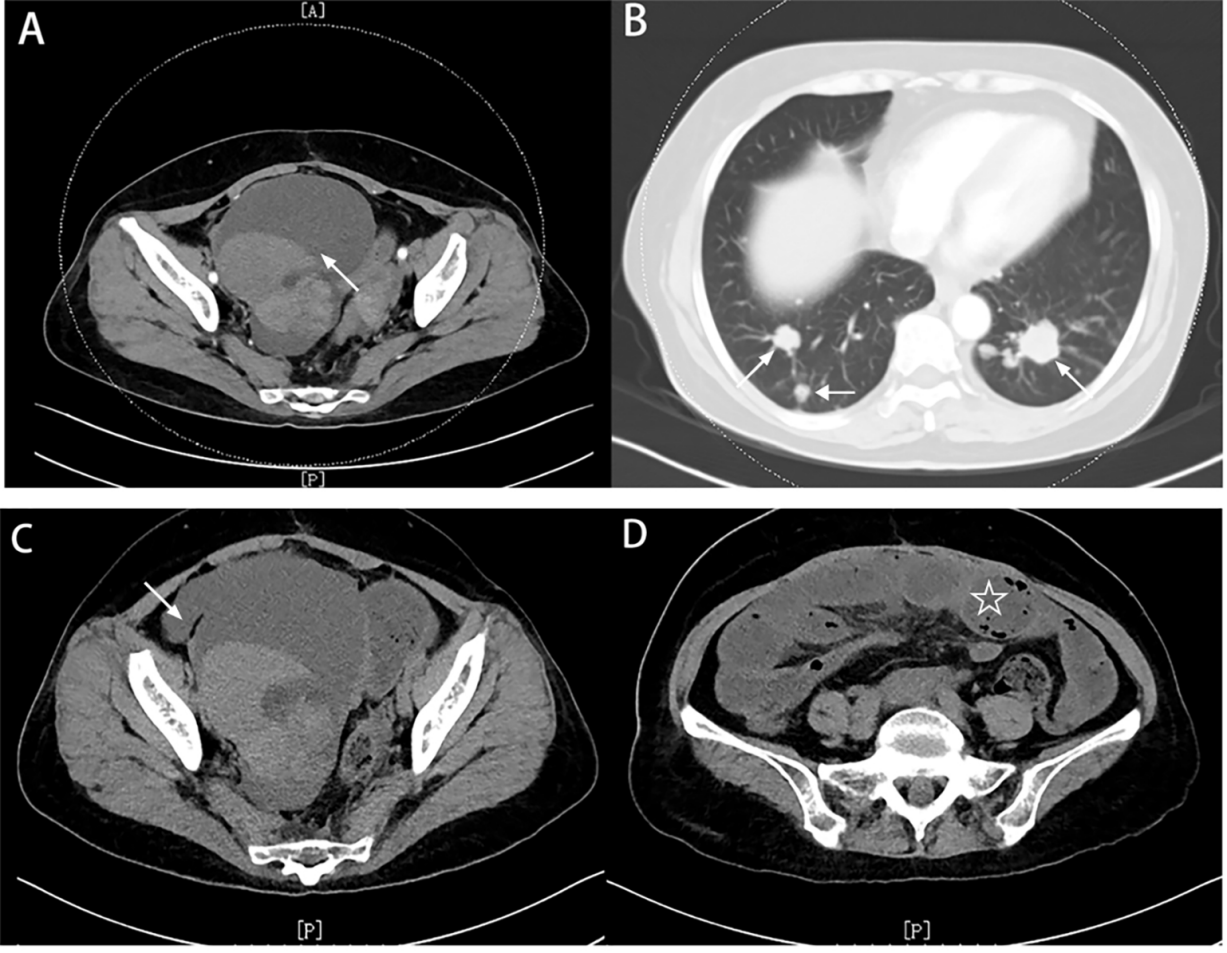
Imaging before the insertion of an ileus tube (A) CT showed that the pelvic lesions (white arrow) had progressed significantly. (B) CT showed that multiple metastatic nodules (white arrow) were located in both lungs. (C) CT showed that the obstruction was located in the terminal ileum that had been invaded by the large pelvic tumor (white arrow). (D) The small bowel became significantly dilated due to the obstruction (white asterisk).
